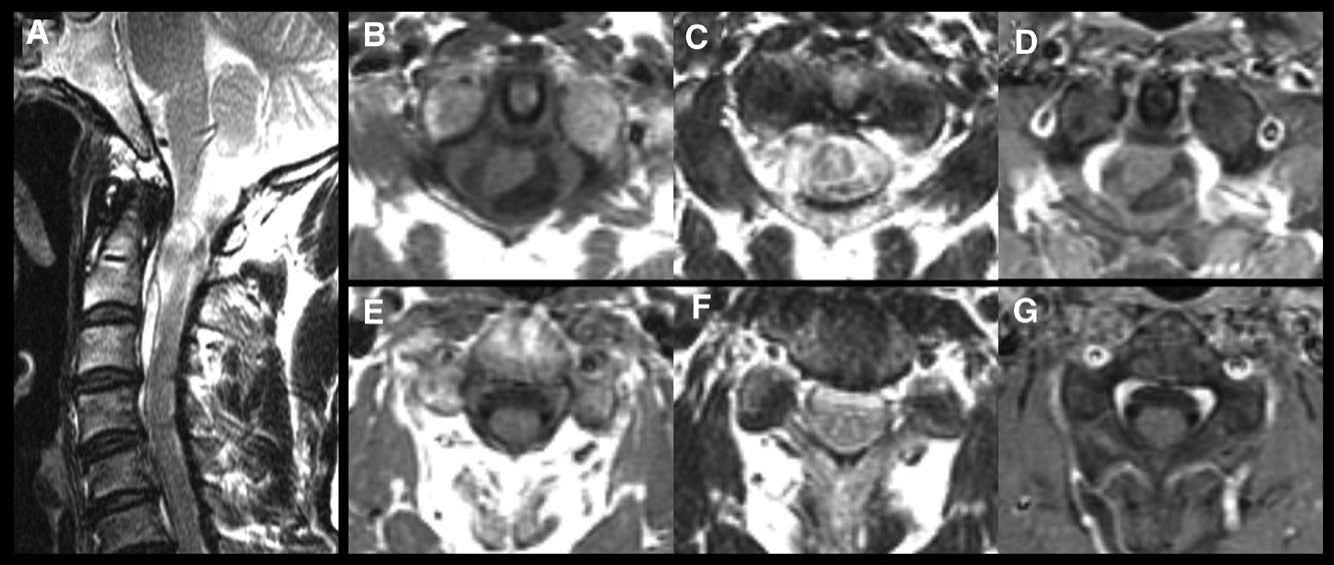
(Case 1) Preoperative spine magnetic resonance imaging (MRI) reveals (A) 2 cysts compressing the spinal cord. The lesions have isointense to low-intensity signals on T1-weighted MRI, high-intensity signal on T2-weighted MRI, and no enhancement after contrast-enhanced MRI at the level of C1–C2 (B–D) and C2–C3 (E–G).
